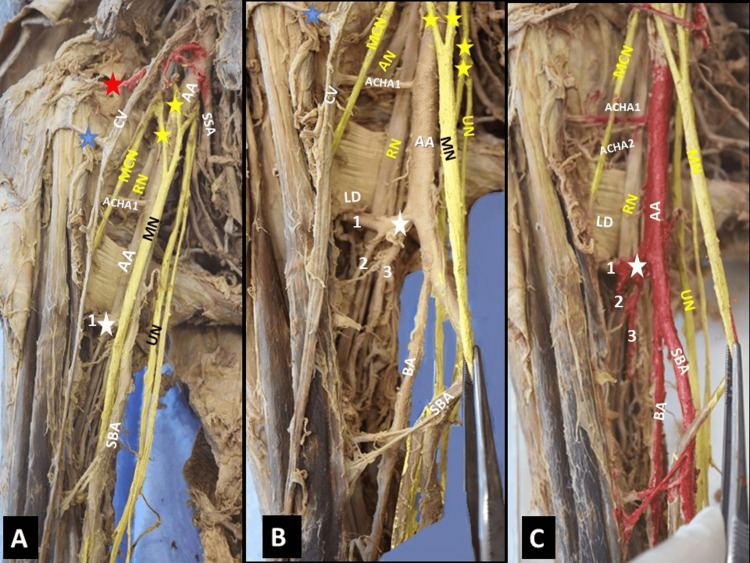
The brachial artery (BA) trifurcation on the right arm, below the tendon of the latissimus dorsi muscle (LD) A. B. The axillary artery (AA) continuation and its trifurcation below the tendon of the LD into a superficial brachial artery (SBA), and the main BA, B. a common trunk (CT) that gave off an aberrant posterior circumflex humeral artery (1), the deep brachial artery (3) accompanied by the radial nerve (RN), muscular branches, and the nutrient humeral artery (2). A. The median nerve (MN) is formed by two lateral roots (yellow asterisks) and a medial root (white asterisk). C. The anterior circumflex humeral artery (ACHA1, ACHA2) duplication. UN-ulnar nerve, AN-axillary nerve, SSA-subscapular artery high origin from the AA second part
